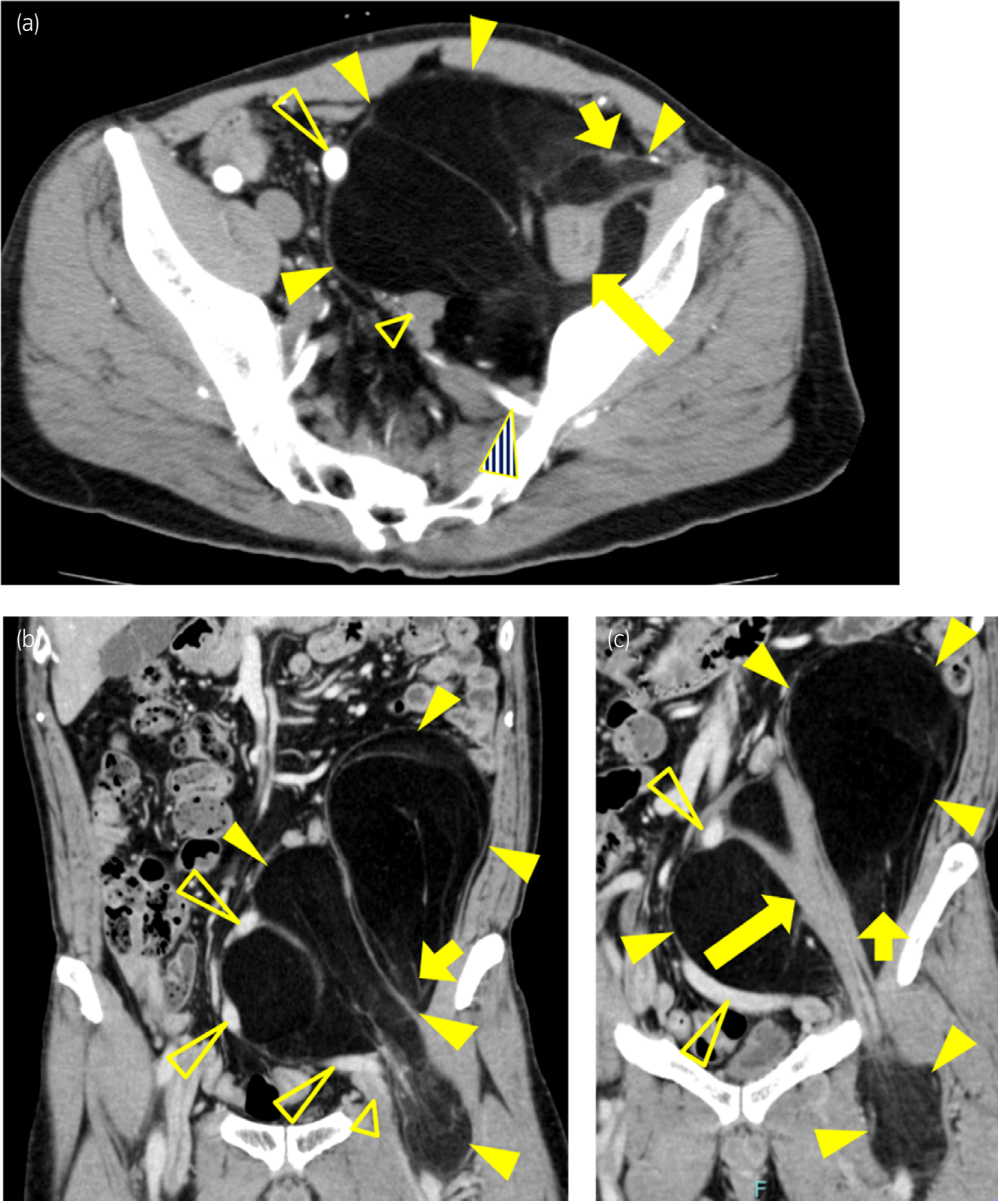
Enhanced CT scan showing a mass occupying the left retroperitoneal and left pelvic cavities extending through the inguinal canal to the thigh. (a) Horizontal section (b, c) Coronal section. Arrowheads, mass; long arrow, psoas major muscle; short arrow, femoral nerve; long open triangle, left external iliac artery; short open triangle, left external iliac vein; triangle with oblique lines, left internal iliac artery.
